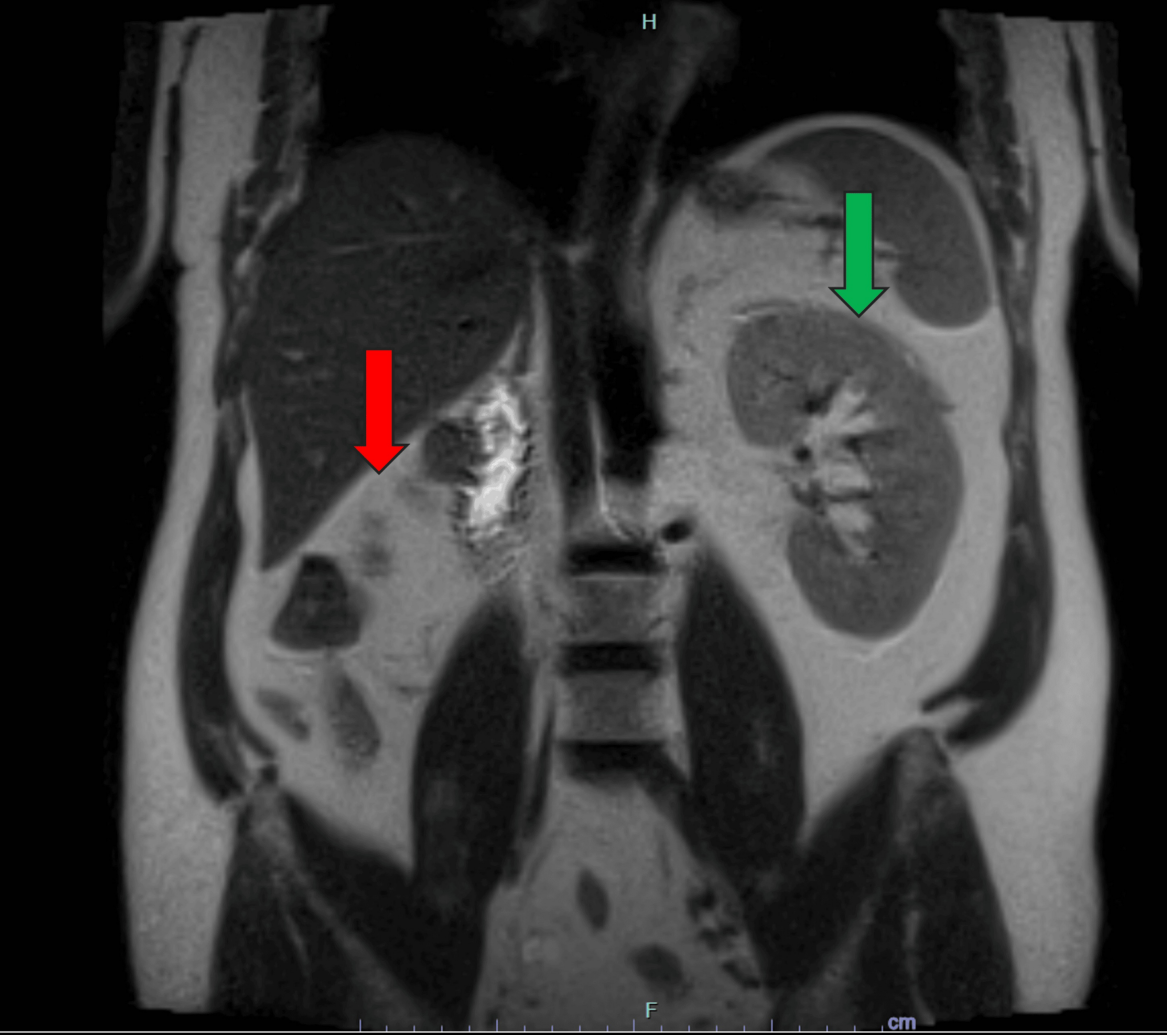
Coronal T2-weighted (T2W) MRI of the upper abdomen demonstrating right renal agenesis (red arrow) and a normal left kidney (green arrow). The image clearly depicts the absence of the right kidney and the normal anatomical appearance of the contralateral kidney. MRI: magnetic resonance imaging
